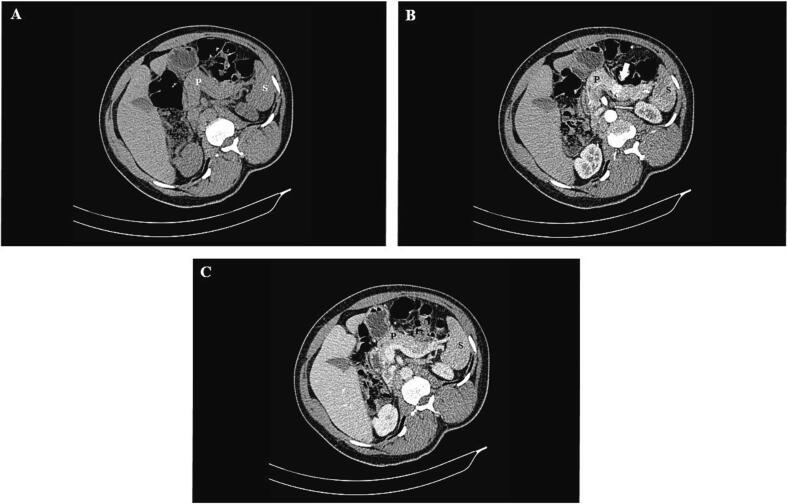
Multiphase abdominal CT scan showing (A) pre-contrast, (B) arterial, and (C) portal venous phases with a hypervascular lesion in the distal pancreas displaying more enhancement during the arterial phase (white arrow in image B). P – pancreas; S – spleen.
